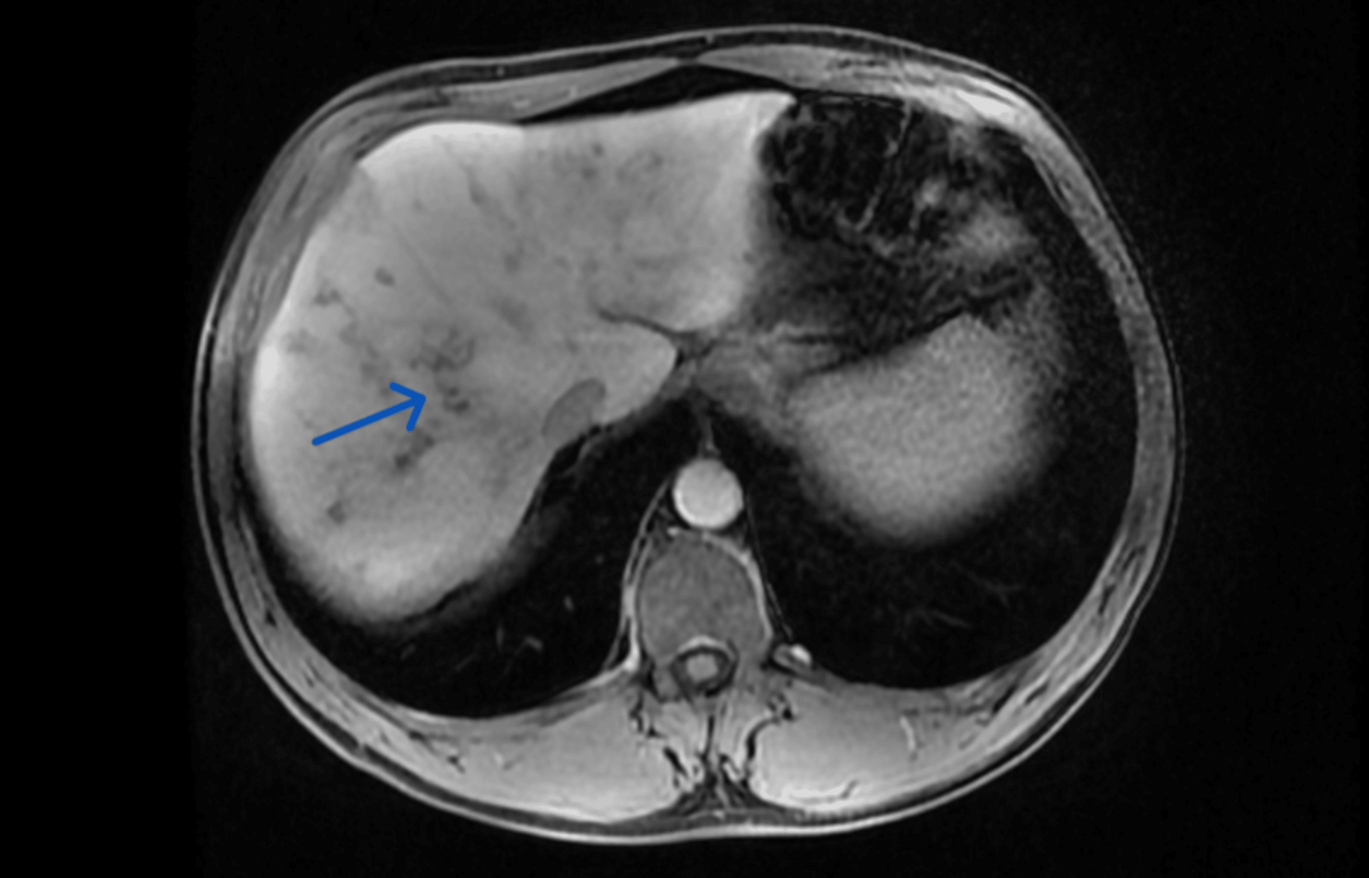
Abdominal MRI performed as part of the diagnostic workup. The abdominal MRI shows several poorly limited pseudonodular hepatic lesions, some of which are confluent, with subcapsular localization. Some lesions present a serpiginous pattern (arrow).
